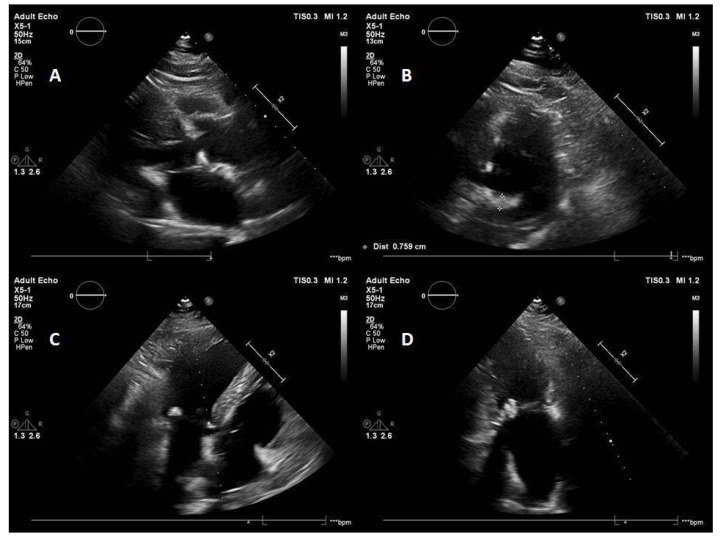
Echocardiographic examination of the patient. Panels (A–D): parasternal long axis and short axis, apical 4-chamber and 2-chamber views showing the conspicuous posterior and mild anterior mitral annular calcification and mild calcification and thickening of the aortic valve. Panel (B): parasternal short axis view at the level of mitral annulus reveals a posterior mitral annular calcification of 8 mm, which exceeds the significant level (>4 mm).
